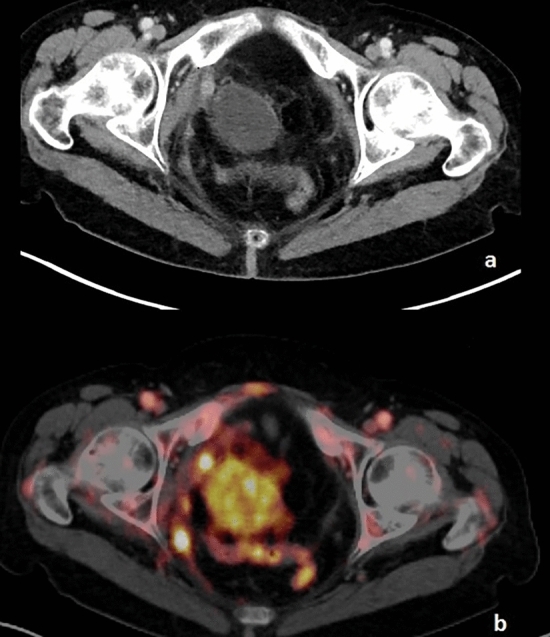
Patient with peritoneal carcinomatosis. a CT image b corresponding PET Image
